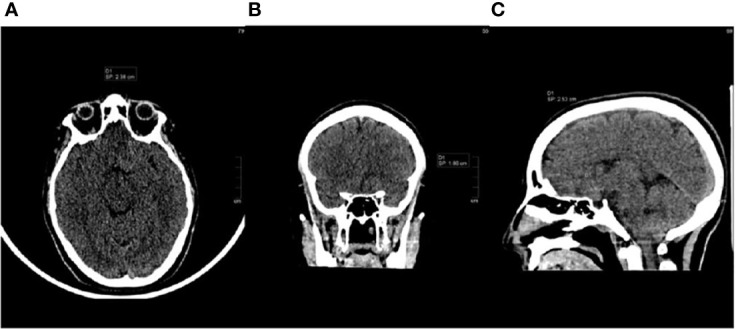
Brain CT scan at diagnosis, August 2016 [(A): axial, (B): coronal, (C): sagittal].
